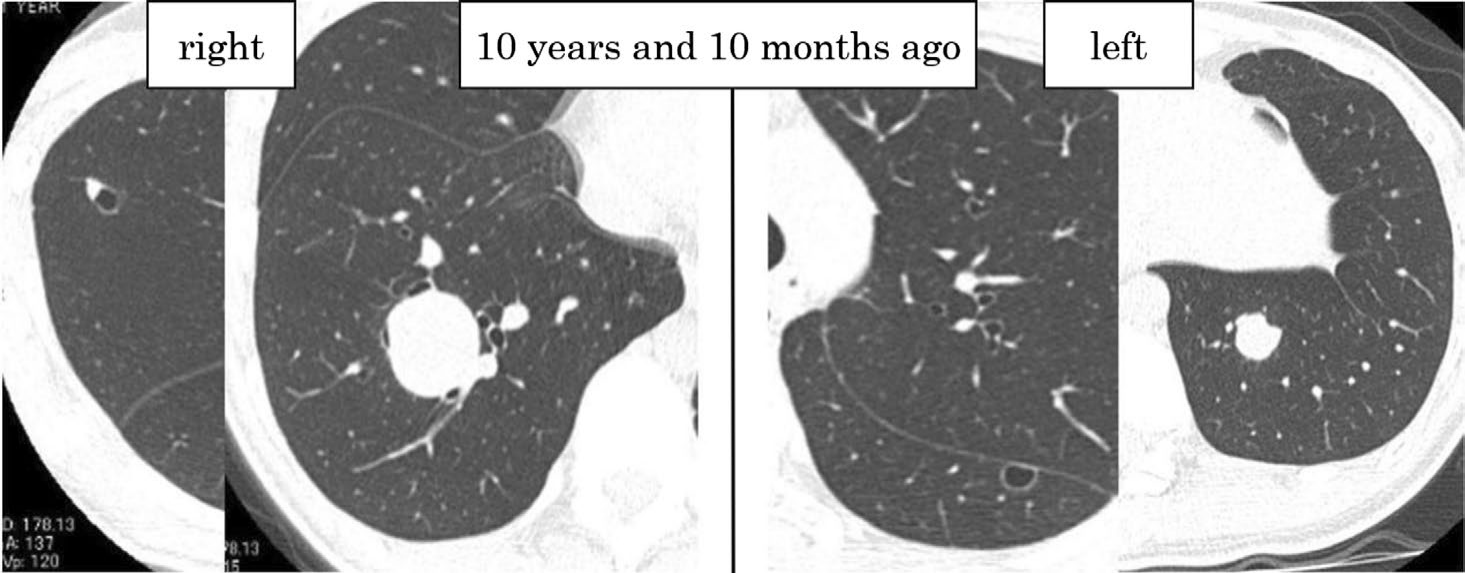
Computed tomography reveals multiple tumors in the right upper, right lower, and left lower lobes after the first metastasectomy.
